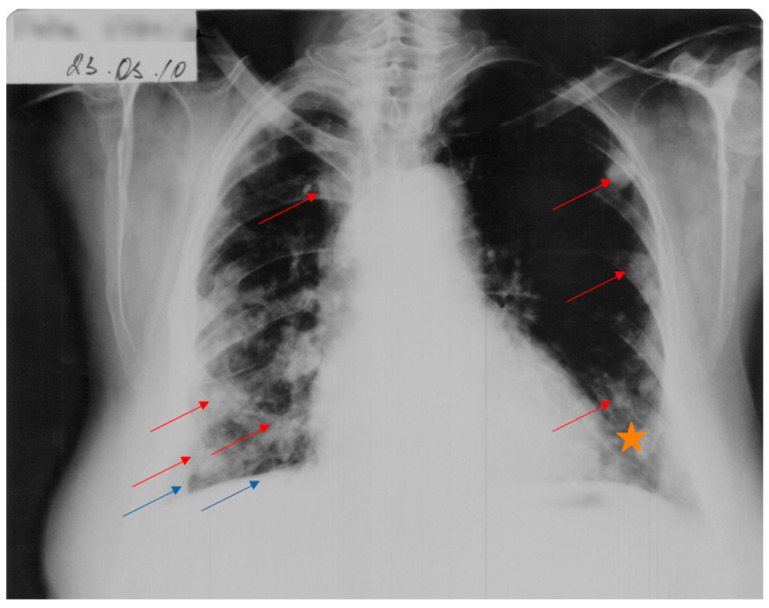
Cardio-thoracic radiograph showing a favorable evolution of the cysts (red arrows) and diffuse para-cardiac opacity (star) under antiparasitic treatment. Persistence of right-lung retraction can be observed (blue arrows).
